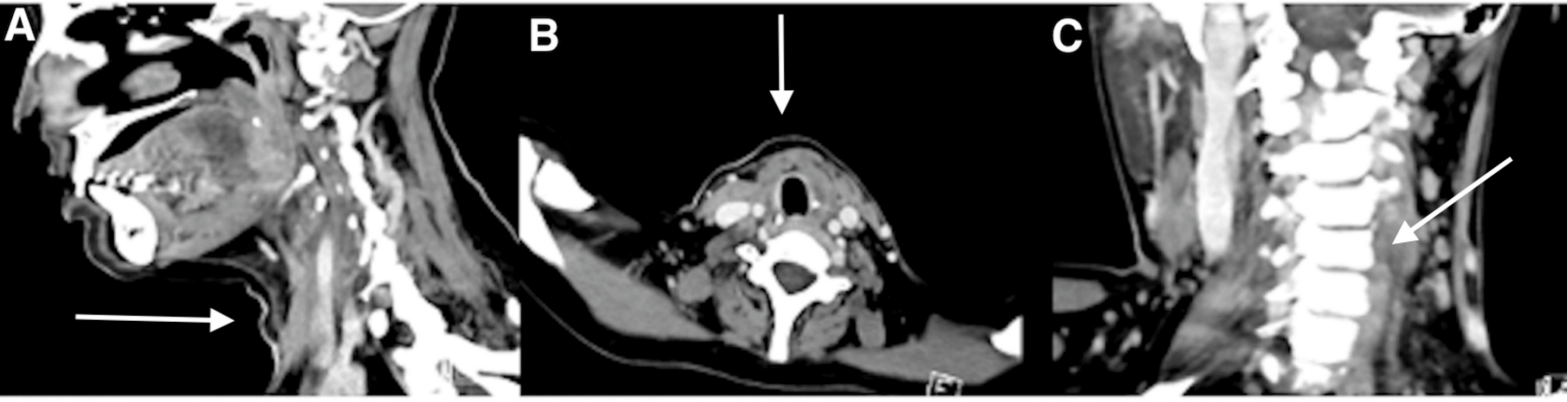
Second computed tomography (CT) of the thyroid post-resection and completion of chemotherapy (A) Sagittal, (B) transverse horizontal, and (C) coronal dimensions on computed tomography (CT) of the anatomical thyroid bed post total thyroidectomy and completion of chemotherapy (arrows).
